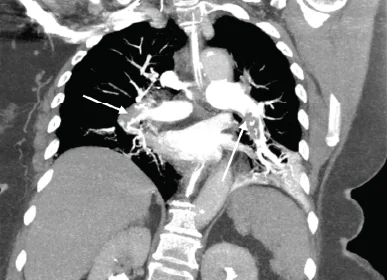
Computed tomography angiography of the chest (coronal view) showing extensive bilateral pulmonary emboli (arrows) in an elderly patient diagnosed with herpes zoster ophthalmicus.
